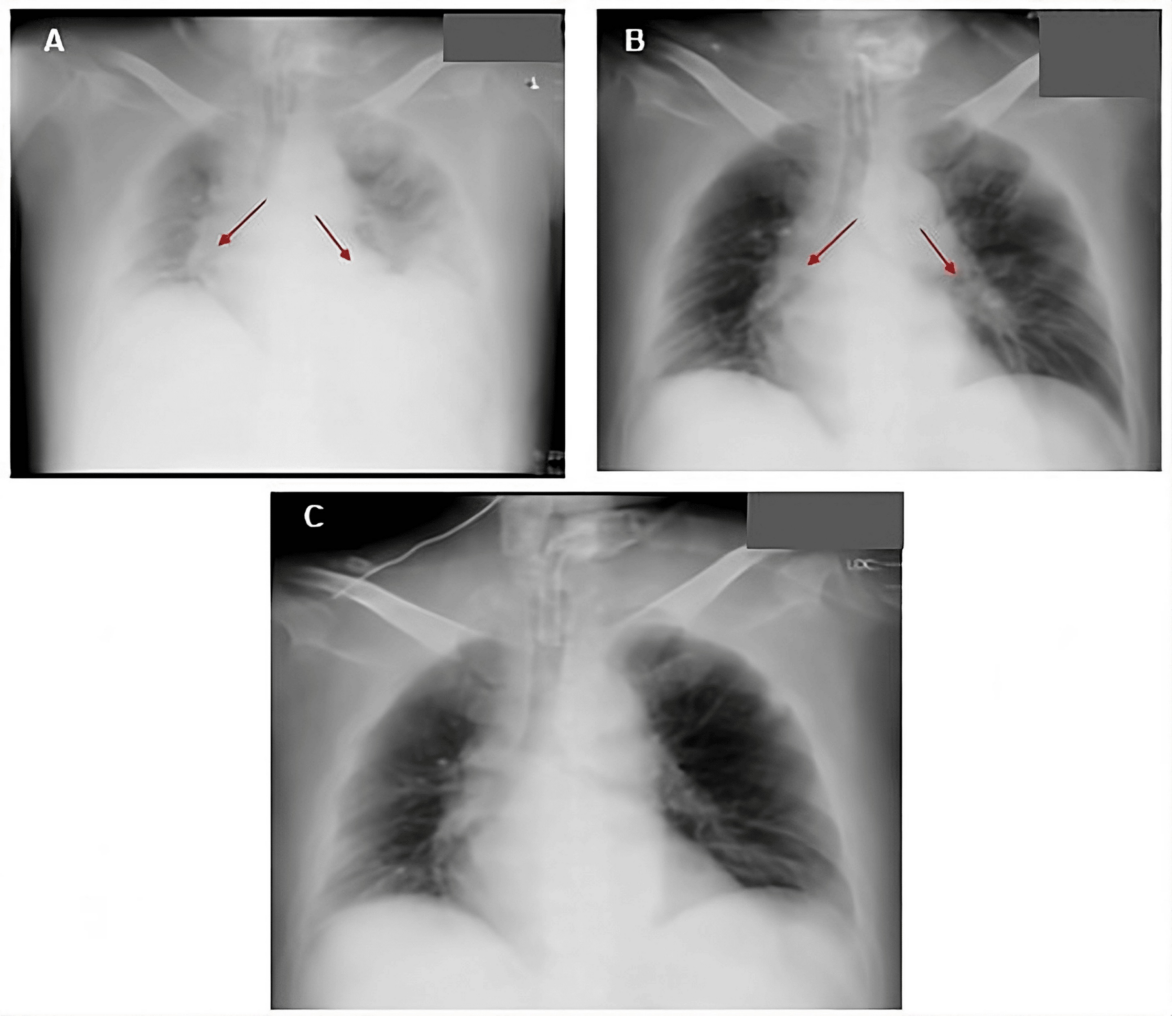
Serial chest radiographs showing progressive resolution of ARDS. (A) Chest radiograph on day 0, showing diffuse bilateral pulmonary opacities consistent with acute respiratory distress syndrome (ARDS) (arrows).
